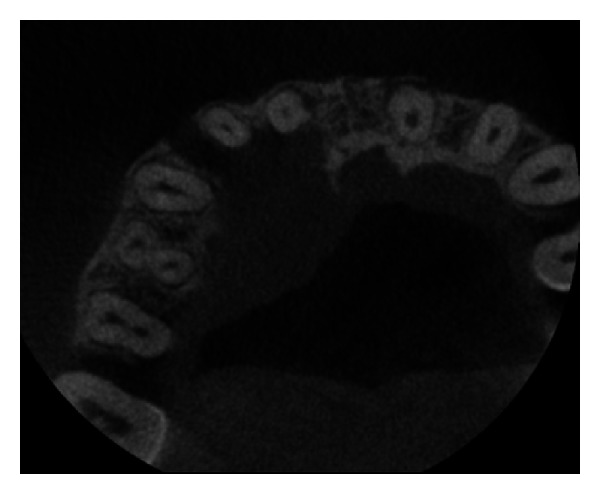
Pre-operative CBCT image in axial plane showing disruption of buccal and palatal cortex.
